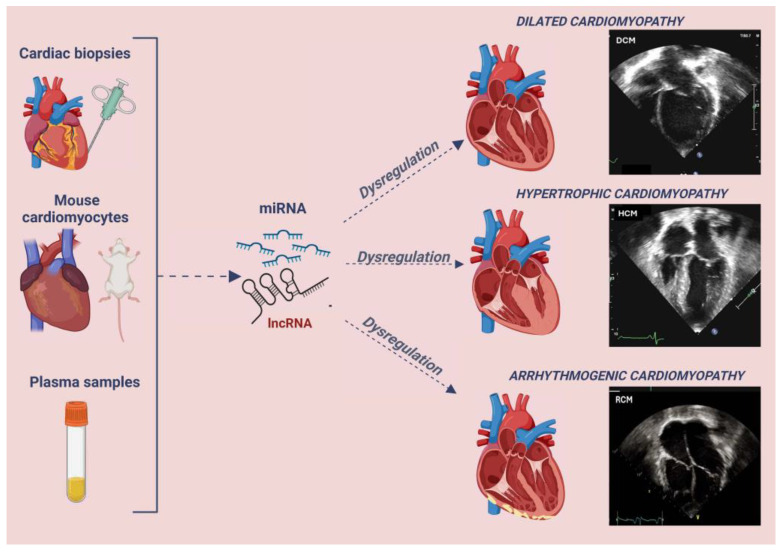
Non-Coding RNAs and their sources in the analyzed studies on cardiomyopathies. Figure created with Biorender; echocardiographic images are part of our archive.
